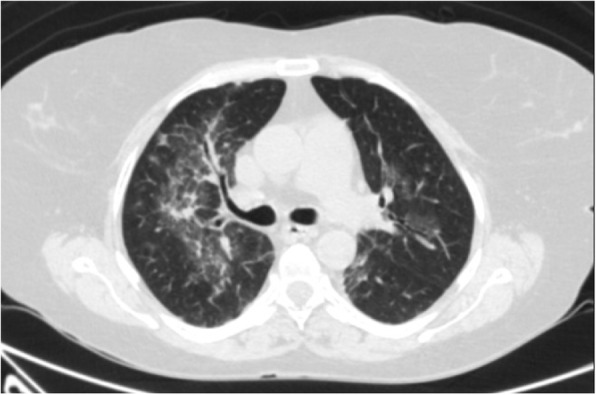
Pneumonia due to P jiroveci infection: thin section CT shows parahilar GGO with reticulation (crazy-paving appearance)
